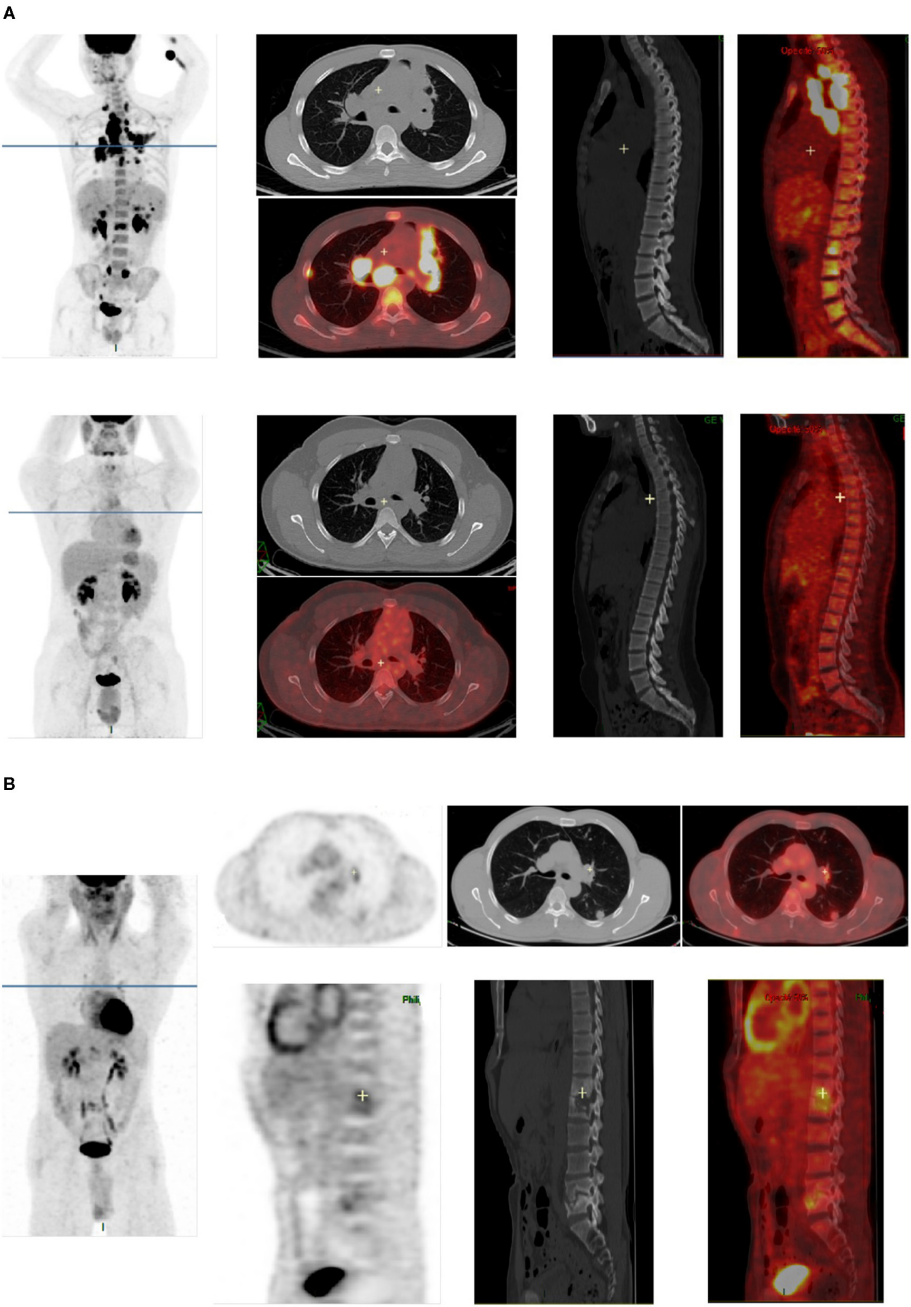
Examples of [^18^F]FDG-PET/CT images in two patients cured at the end of treatment. (A) PET1 and PET2 images of a patient cured at the end of treatment. All abnormalities seen on PET1 (lung lesions, mediastinal lymph nodes, bone lesions in dorsal and lumbar spine) completely disappeared on PET2. (B) PET2 images of a patient cured at the end of antibiotherapy, showing residual FDG uptake in cured lesions [left lung, left hilar lymph node, and spine (T12, L1, and L5)].
